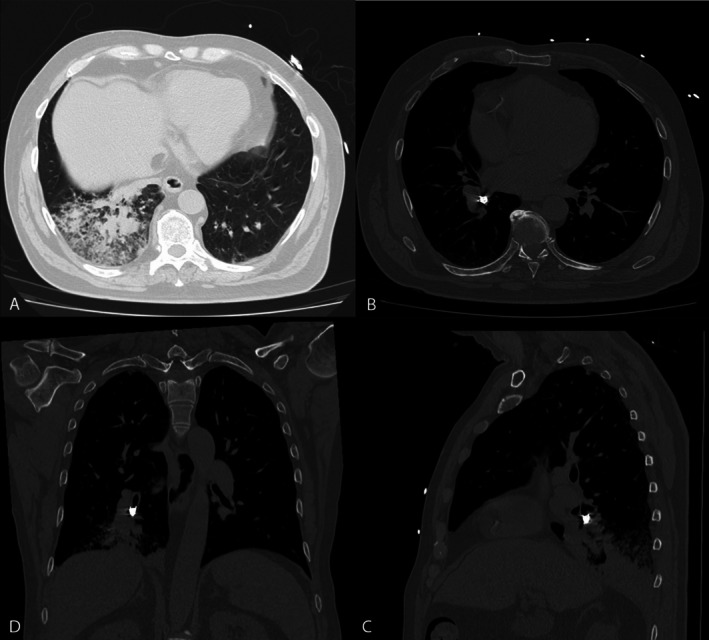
(A–D clockwise from top left): Computed tomography of the chest demonstrating a lucency within the right lower lobe bronchus with associated distal consolidation and partial right lower lobe collapse. Metallic artefact secondary to a dental crown is noted.
